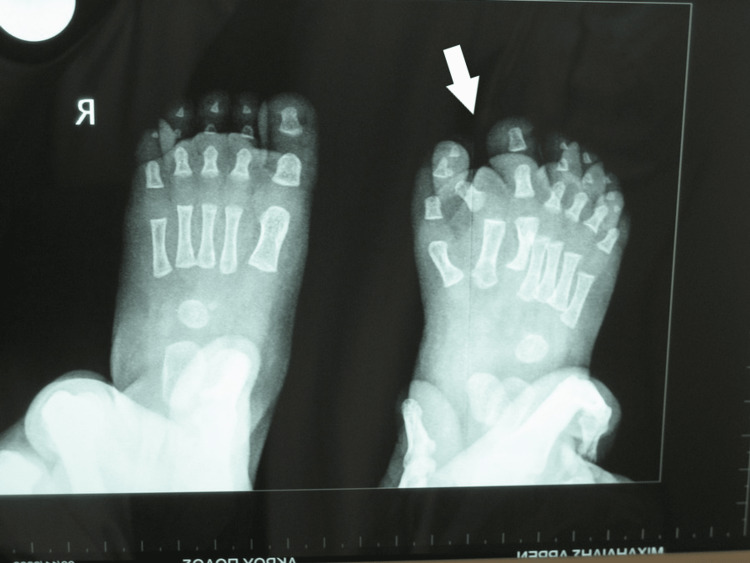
X-ray of the third patient's affected foot. X-ray of the foot with the 3d ray with a toe with two phalanges. The medial supernumerary toes, first, and second appeared enlarged and with a deviation of the axis. The remaining 4-7 metatarsals and toes on the lateral side appeared with normal shape. Cuneiforms and tarsal bones appeared normal on the initial X-ray.
